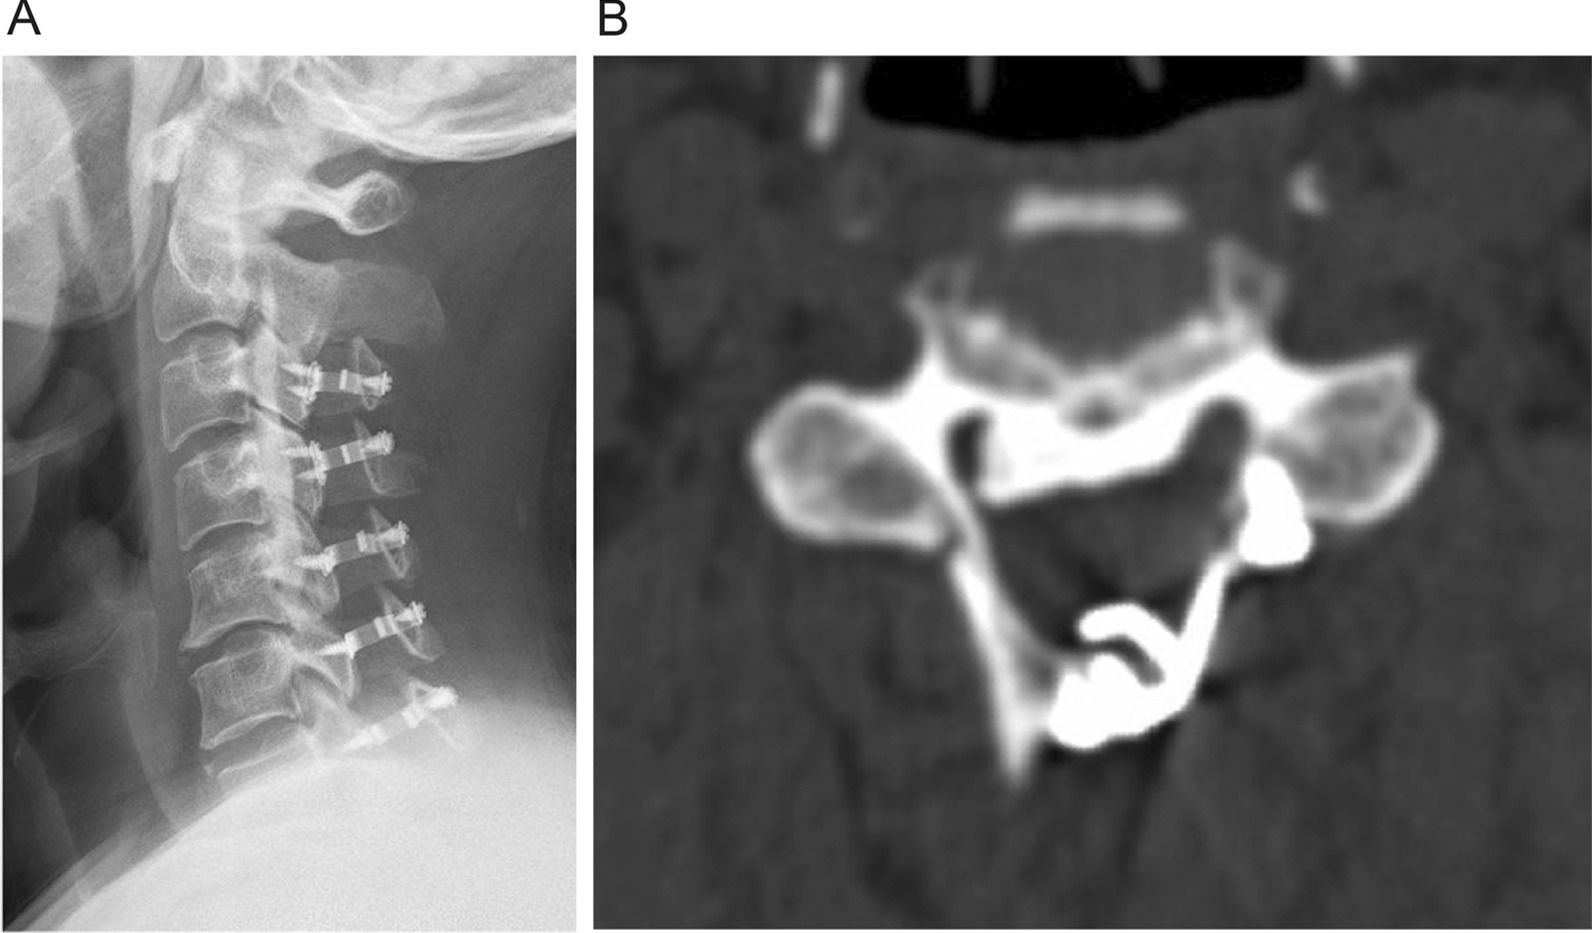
A and B Lateral X-ray and axial computed tomography slice of patients with C-OPLL undergoing ODL. The laminas were opened from one side, and the gaps were fixed with arch plates (C3–7)
